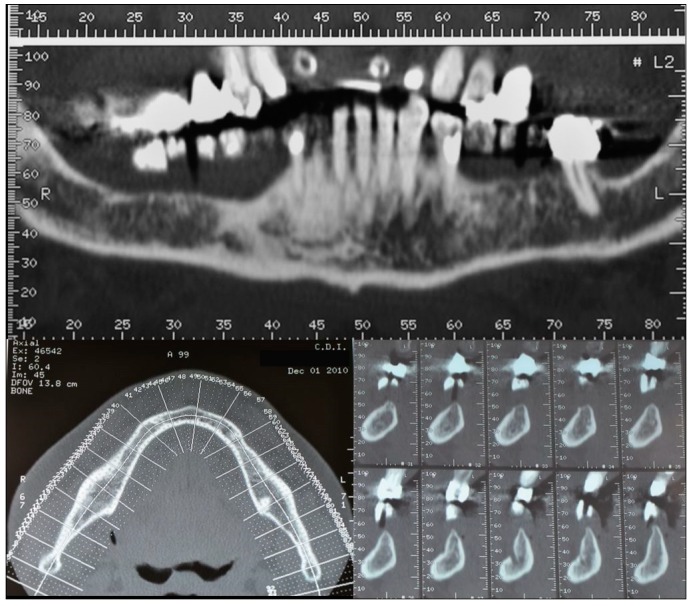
CT scans showing the horizontal atrophy in the lower right quadrant.
